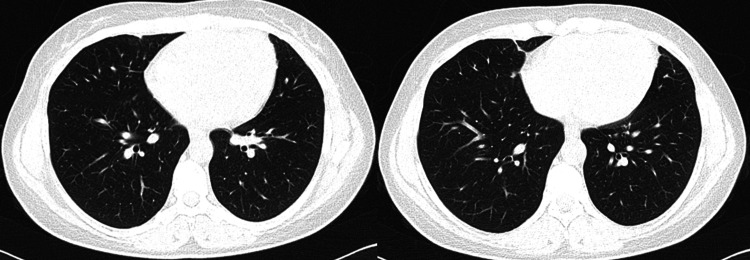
CT after six months without parenchymal densification
